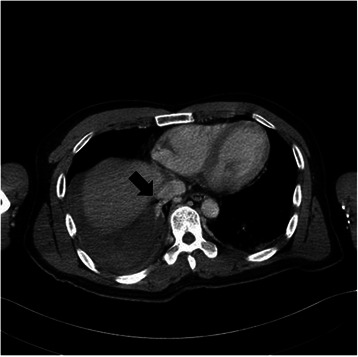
Chest CT shows that a large amount of hemothorax and an active bleeding, presumably from the posterior intercostal or the phrenic artery, with focal aneurysmal changes. In addition, no injuries to the thoracic organs, including ribs, the lung, the mediastinum, or the diaphragms, are observed.
